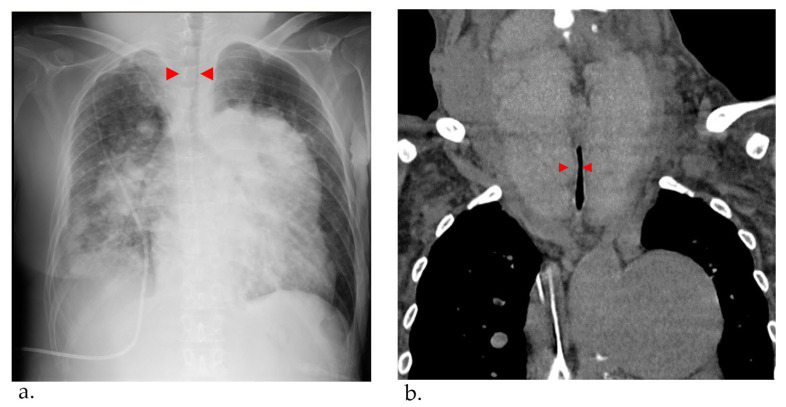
(a) Chest X-ray pulmonary congestion, enlargement of the cardiac shadow, and airway stenosis were detected (red arrowhead). (b) Computed tomography Thyroid gland enlargement was observed. Tracheal compression and stenosis due to goiter (red arrowhead).
